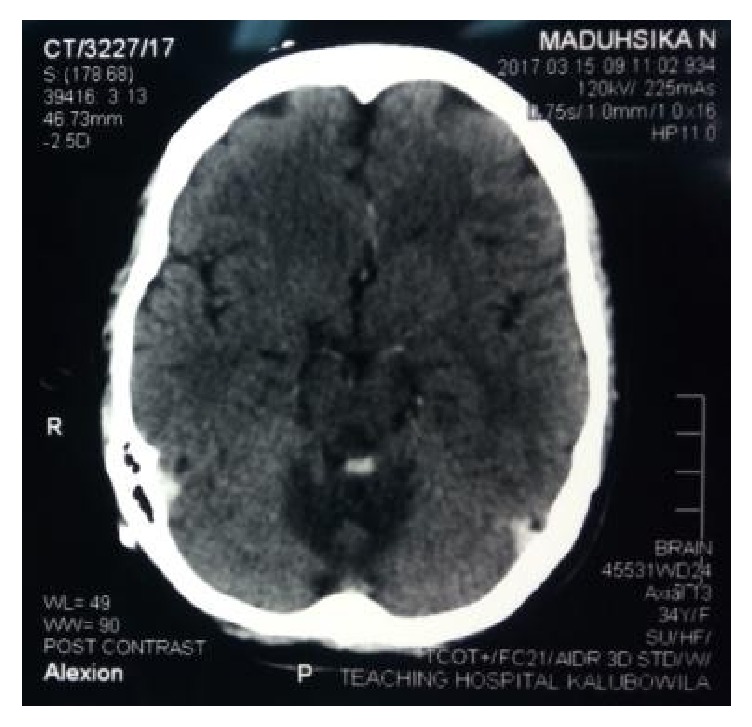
Noncontrast CT brain showed early hydrocephalus with a suspicion of a posterior fossa space occupying lesion.
